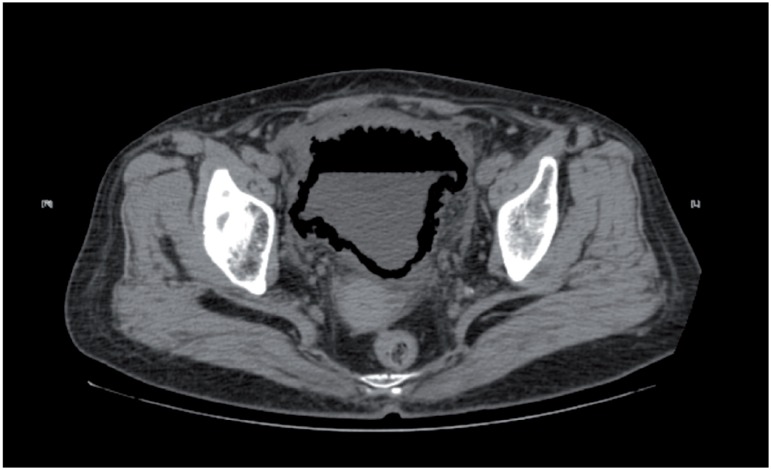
A prominent air-fluid level within the bladder on computed tomography.
